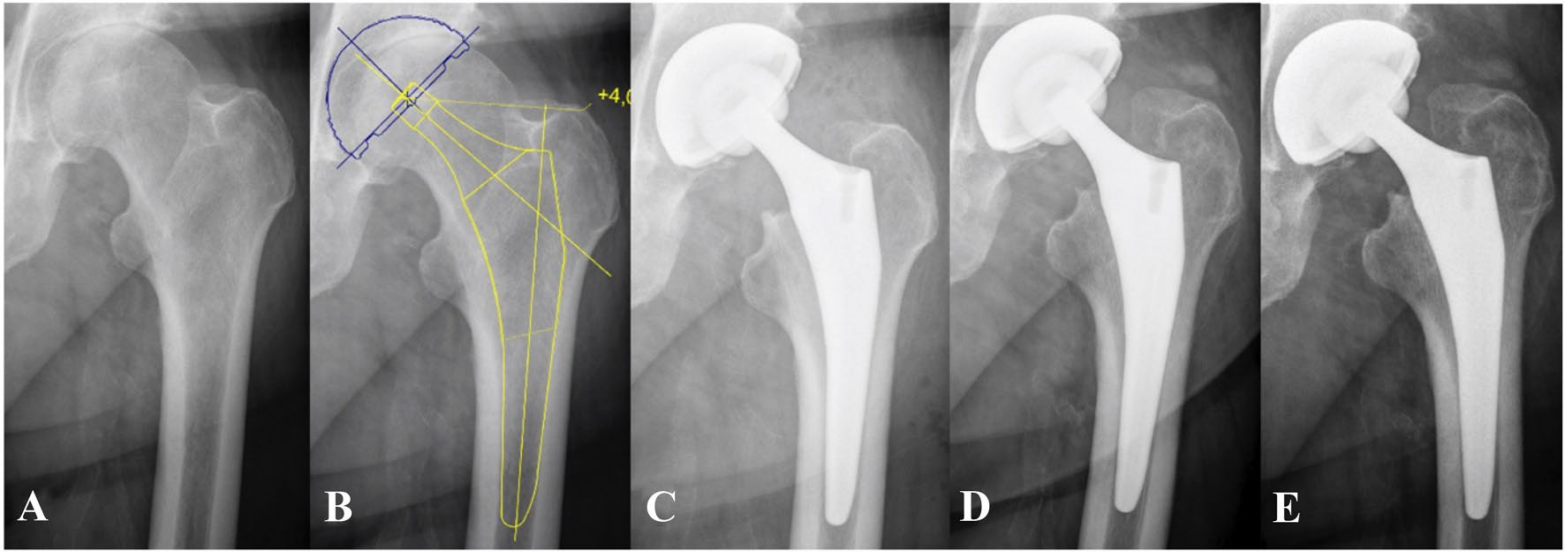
The X-ray series presents the procedure of the patient with the largest detected subsidence A preoperative situation showing osteoarthritis of the hip B preoperative prosthetic planning C immediate postoperative X-ray D subsidence of 5.3 mm was detected by EBRA-FCA 6 weeks after surgery E subsidence of 12 mm was detected by EBRA-FCA 12 months after surgery
